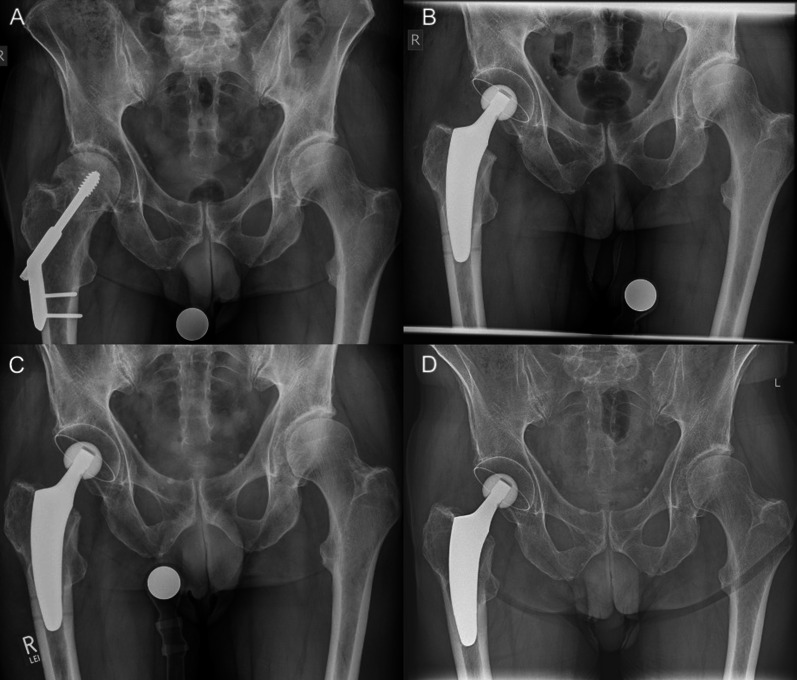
Radiographs of a 73-year-old male patient with hardware failure: A preoperative; B postoperative with fit-and-fill fixation; C 6-week follow-up; D 49-month follow-up (no signs of loosening or fracture)
